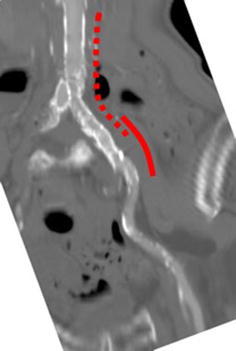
Computed tomography findings. There was no limb stenosis or kinking after endovascular aneurysm repair (*dotted line* left graft limb, *solid line*, SMART^®^ stent)
